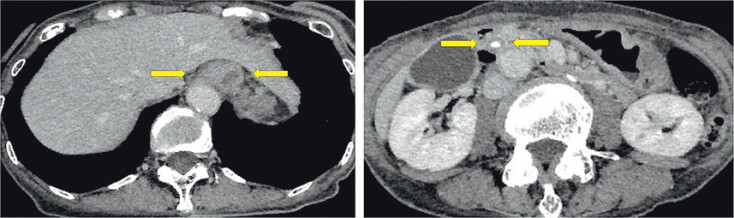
Portal phase of contrast-enhanced CT revealed wall thickening
